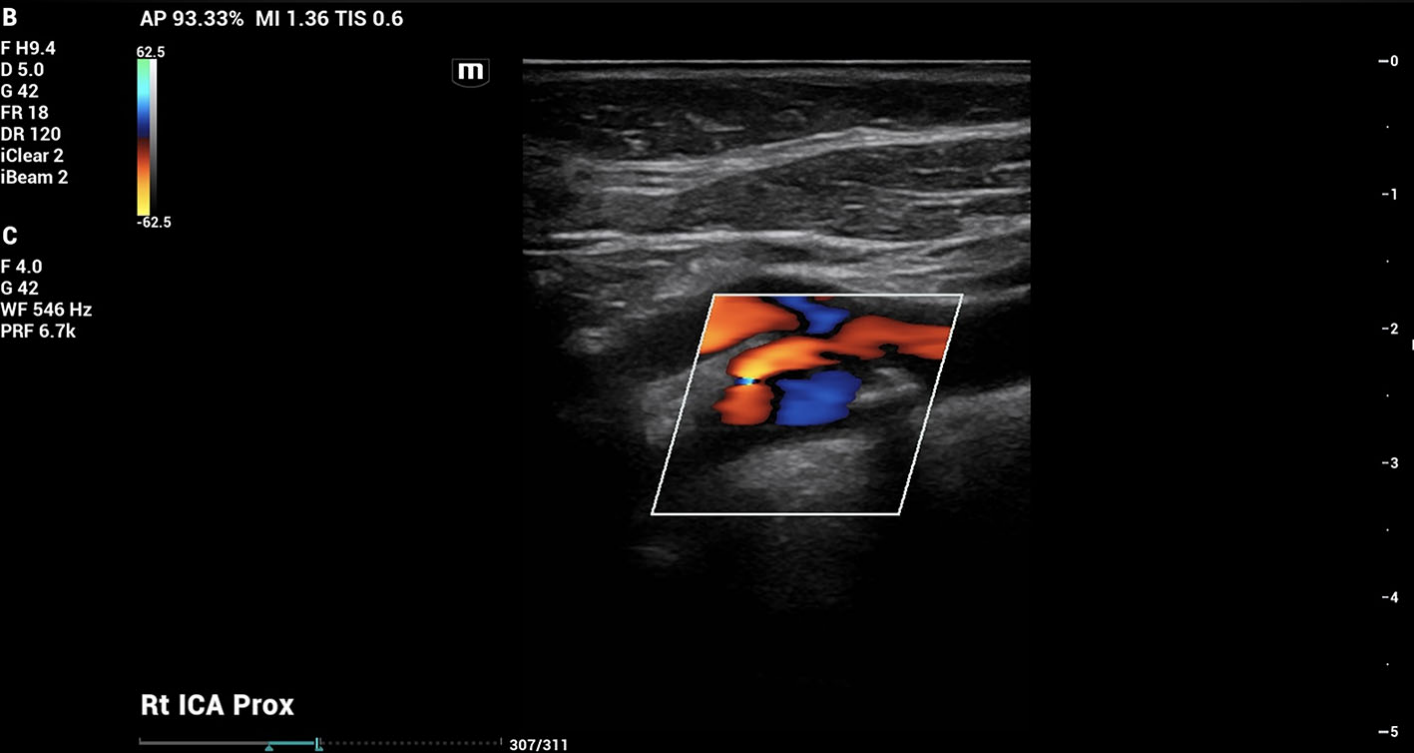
Carotid doppler imaging. Carotid doppler showed 50% stenosis in right carotid bulb and proximal cervical internal carotid artery (ICA).
